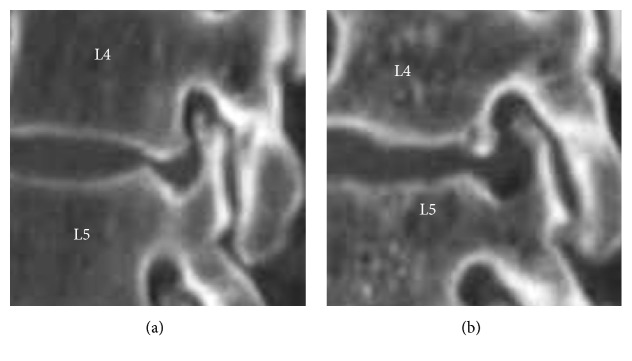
CT images of SCOT. (a) preoperative; (b) postoperative.
